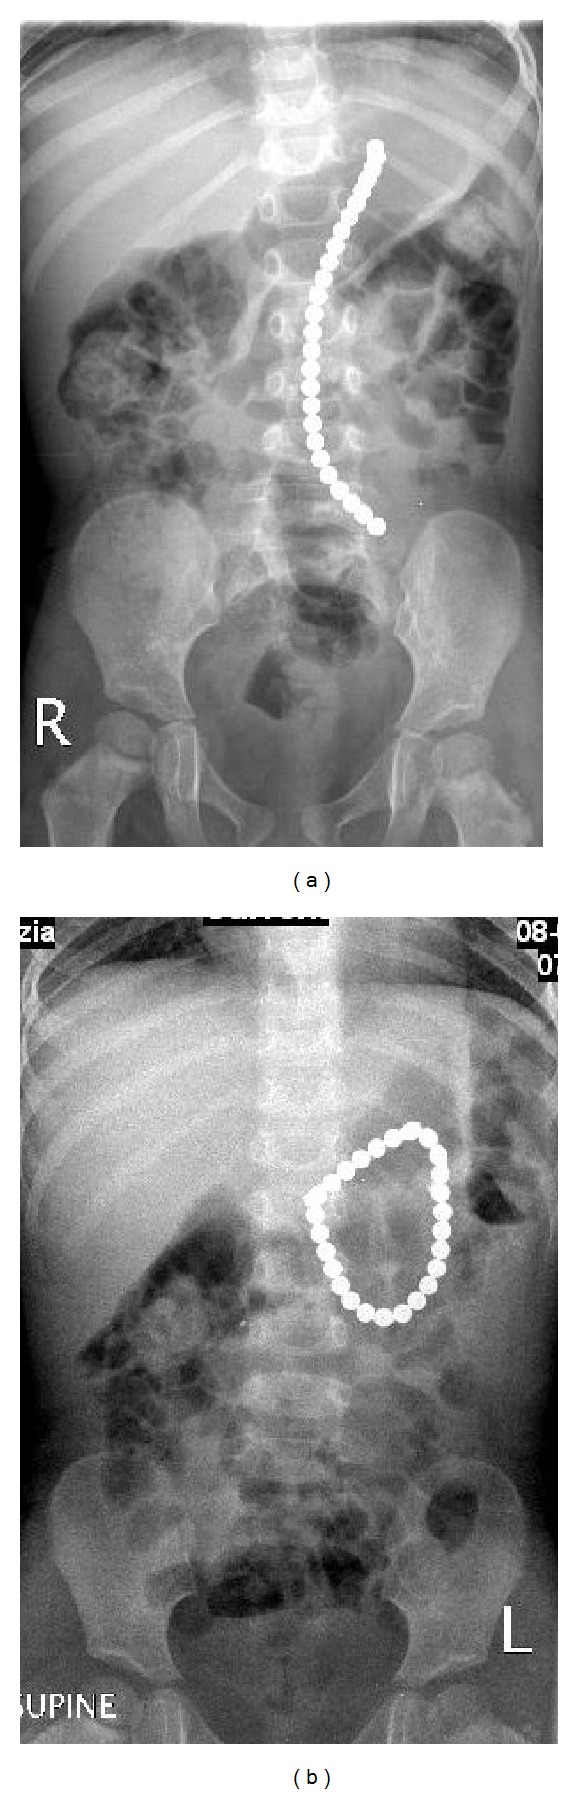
(a) Plain X-ray of abdomen of 2-year-old girl showing ingested 26 pieces of rare-earth magnets joined together in linear fashion. (b) Repeated plain abdominal X-ray of the same patient after 36 hours showing ingested rare-earth magnets forming a ring in the left upper abdomen with no free air and/or obstructive bowel pattern.
